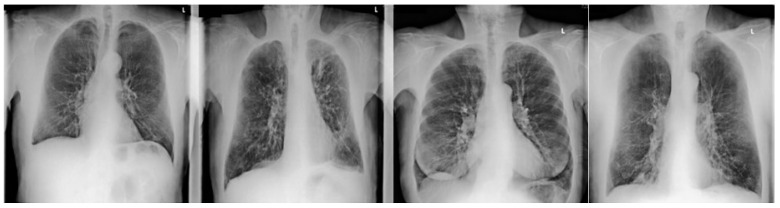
Dual energy soft tissue x-ray image of non-mild-moderate-severe emphysema in the right upper lobe.
